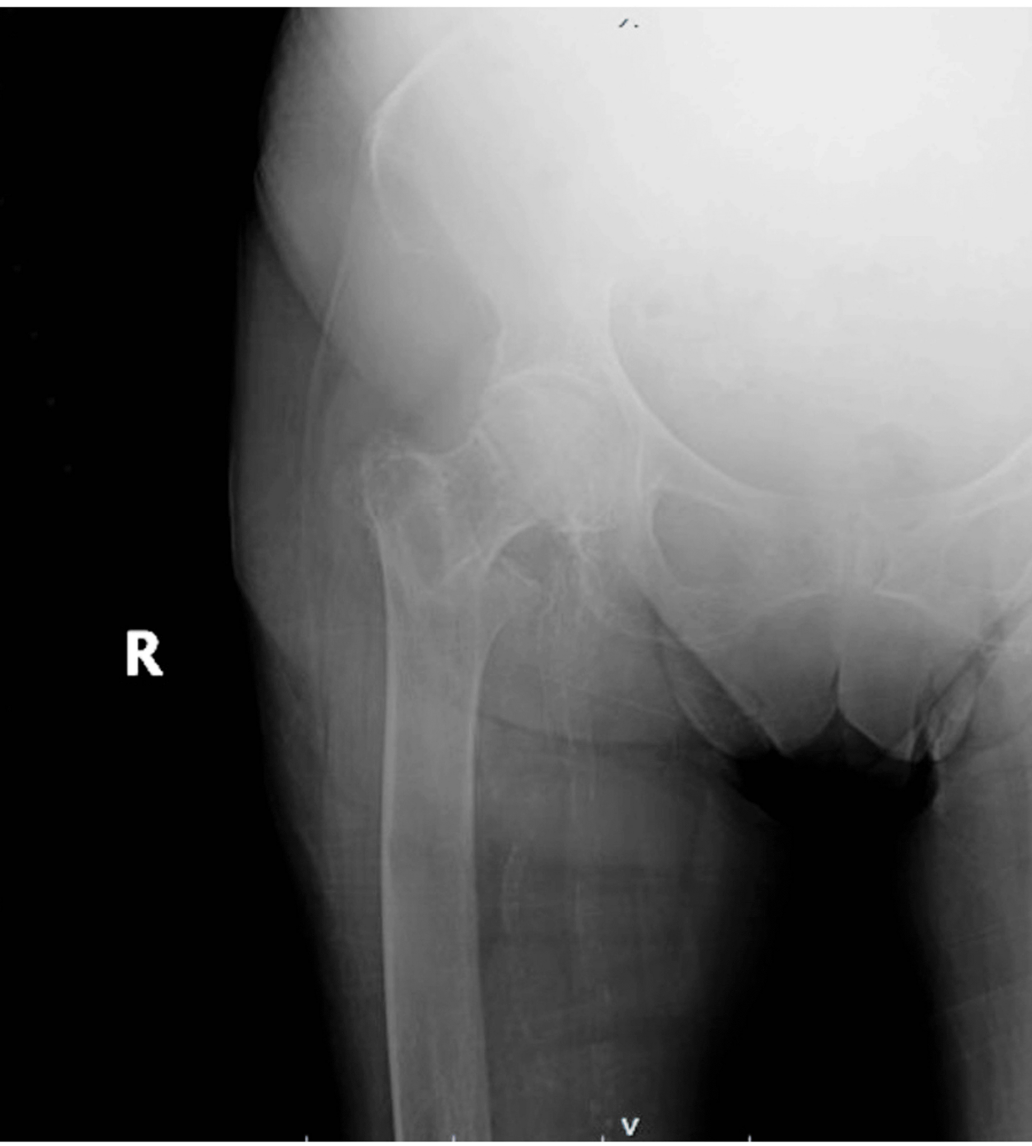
Initial injury anteroposterior (AP) radiograph showing an intertrochanteric fracture of the right hip with generalized osteopenia
